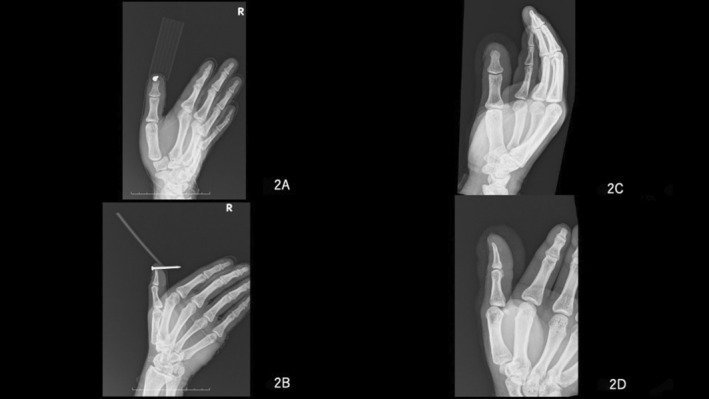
Orthogonal radiographic assessment of a nail‐gun injury to the right thumb (A) Anteroposterior radiograph demonstrating penetration of the thumb by a nail without obvious involvement of the distal phalanx. (B) Lateral radiograph showing the trajectory of the nail through the soft tissue and confirming the absence of distal interphalangeal joint penetration. (C) Postextraction lateral radiograph demonstrating complete removal of the nail without retained radiopaque foreign bodies. (D) Postremoval anteroposterior radiograph demonstrating no fracture or osseous injury of the distal phalanx.
